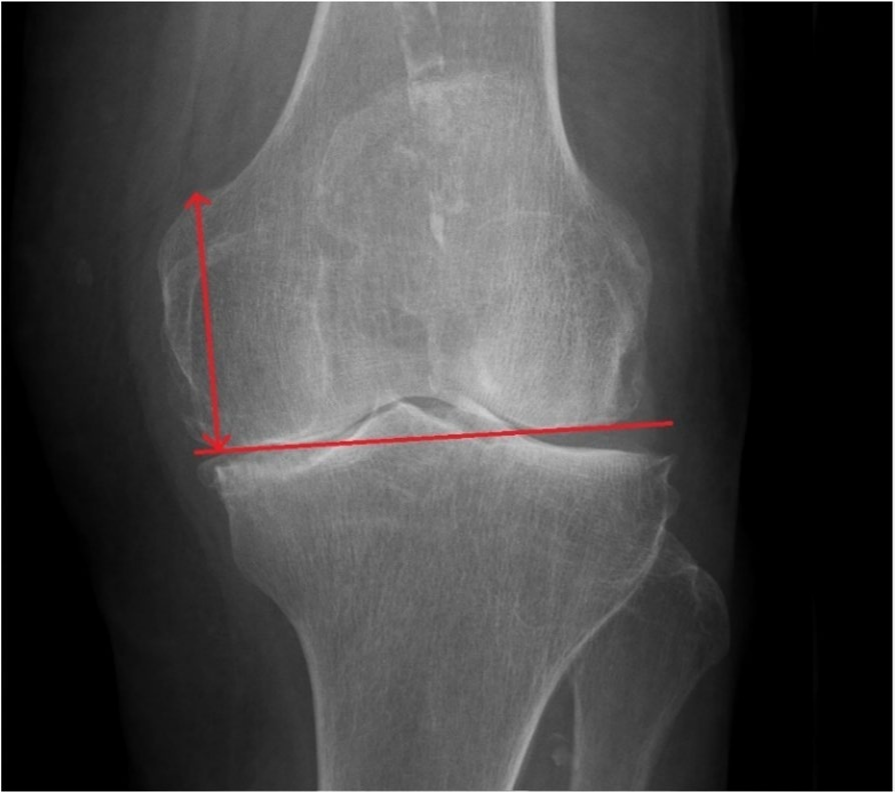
Measurement of preoperative joint line level on the weight-bearing antero-posterior x-ray
